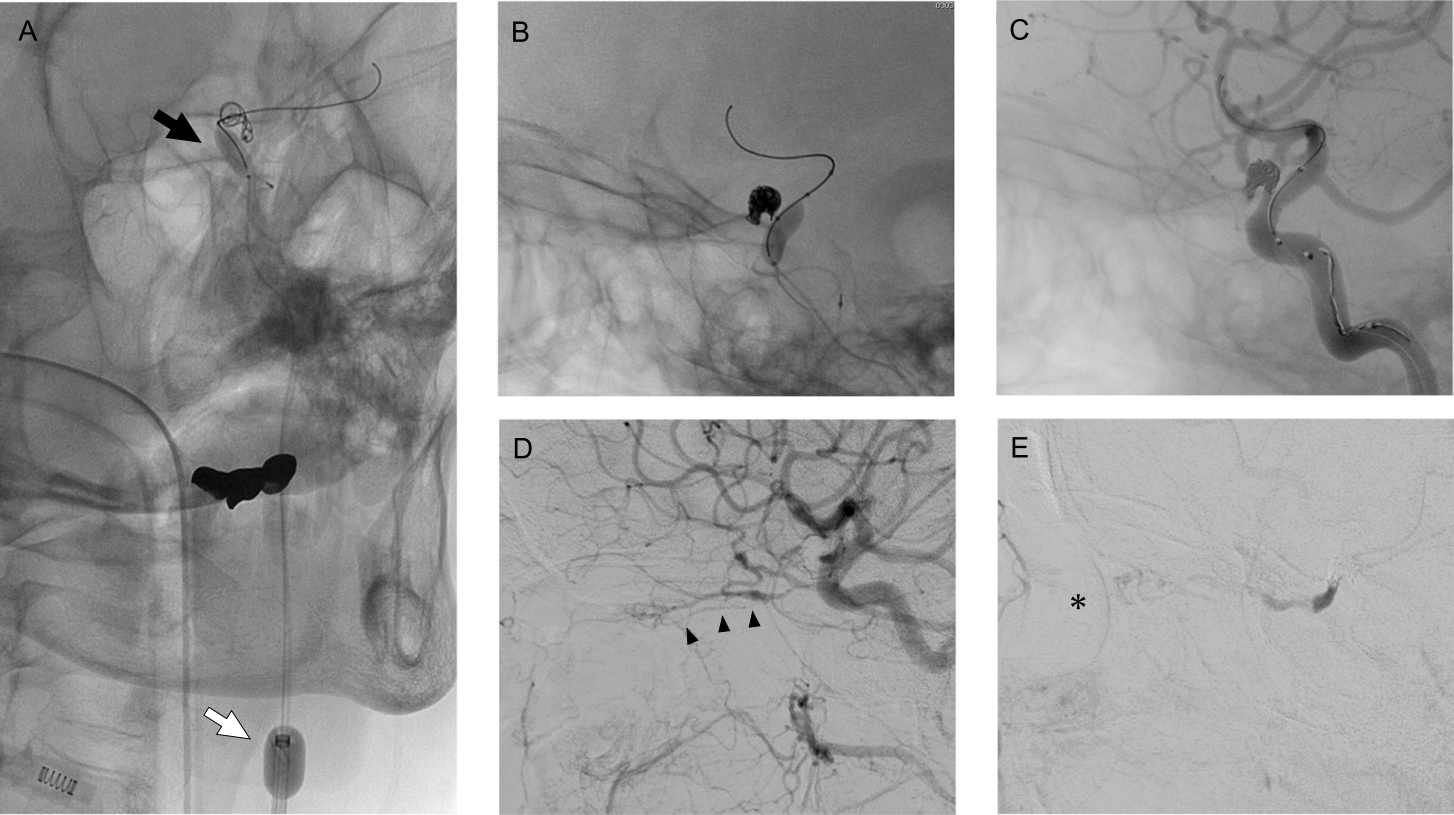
Images of intraoperative cerebral angiography. Right oblique projection of the radiographic image showed double-balloon flow restoration with a balloon-guided catheter placed at the internal carotid artery (ICA) (white arrow) and balloon catheter at the ICA-ophthalmic artery (OA) bifurcation (black arrow). Moreover, the framing coil was placed from the microcatheter in the OA (A). Lateral projection of the radiographic image (B) and left internal carotid arteriography (C) revealed that the compact coil mass was finally placed at the short segment at the origin of the OA. Lateral projection of the common carotid arteriography showed no prograde flow from the ICA into the OA, and the proximal segment of the OA and the central retinal artery (black arrowhead) (D) were retrogradely supplied from the recurrent meningeal artery from the middle meningeal artery. Further, the retinal crescent (asterisk) was detected (E)
